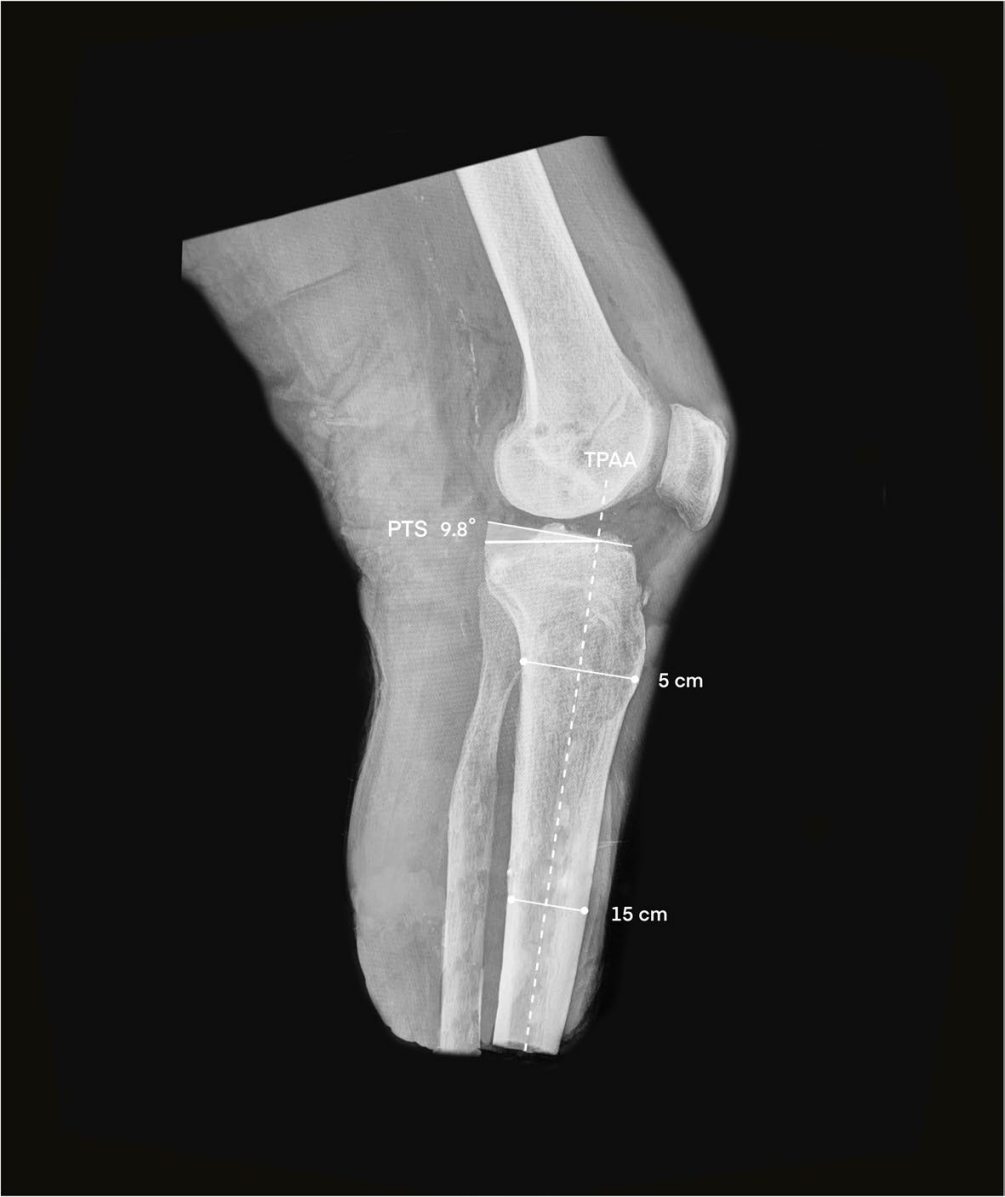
Tibial slope measurement on lateral radiographs. The tibial proximal anatomic axis (TPAA) is drawn connecting the midpoints between the anterior and posterior tibial cortex at five and 15 cm distances to the joint line. A reference line is drawn perpendicular to this at the level of the tibiofemoral joint. The mean between the inclinations of both plateaus is also shown. The angle of this line to the reference line is defined as the posterior tibial slope (PTS)
